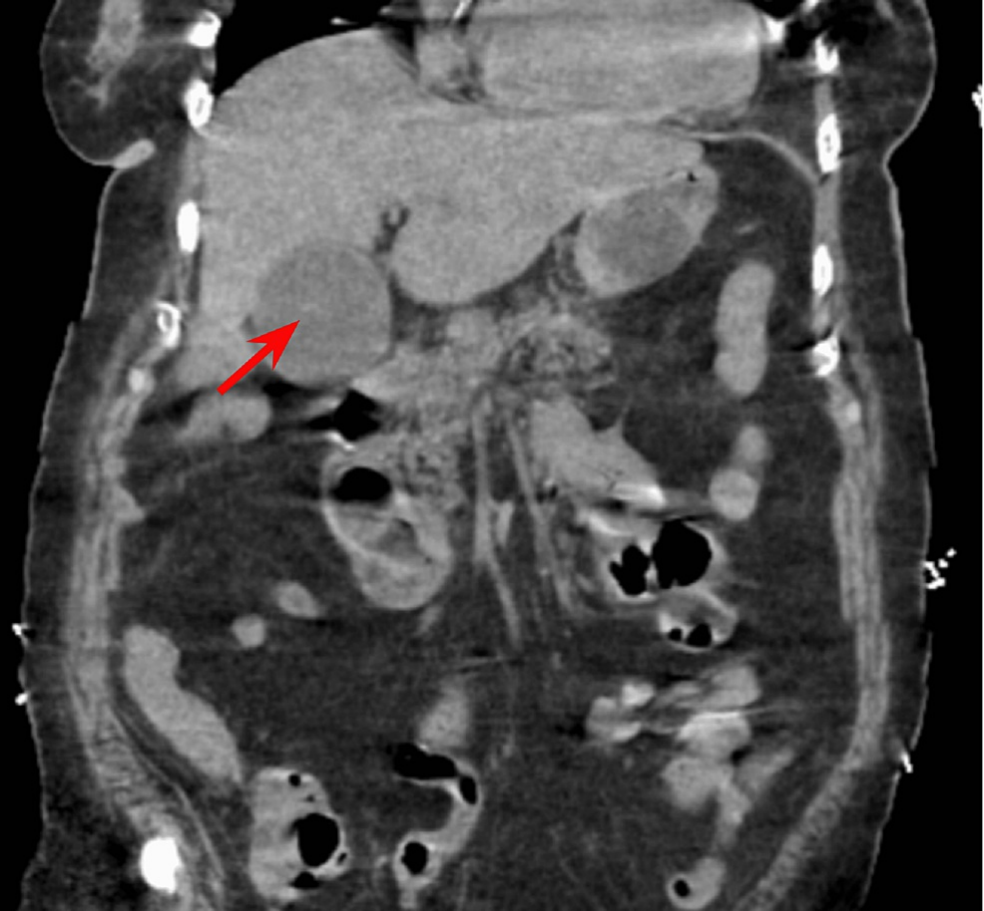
Initial CT abdomen and pelvis in the coronal view CT abdomen and pelvis in the coronal view with narrowing of the window shows intra-luminal membrane in the gallbladder (solid arrow).
